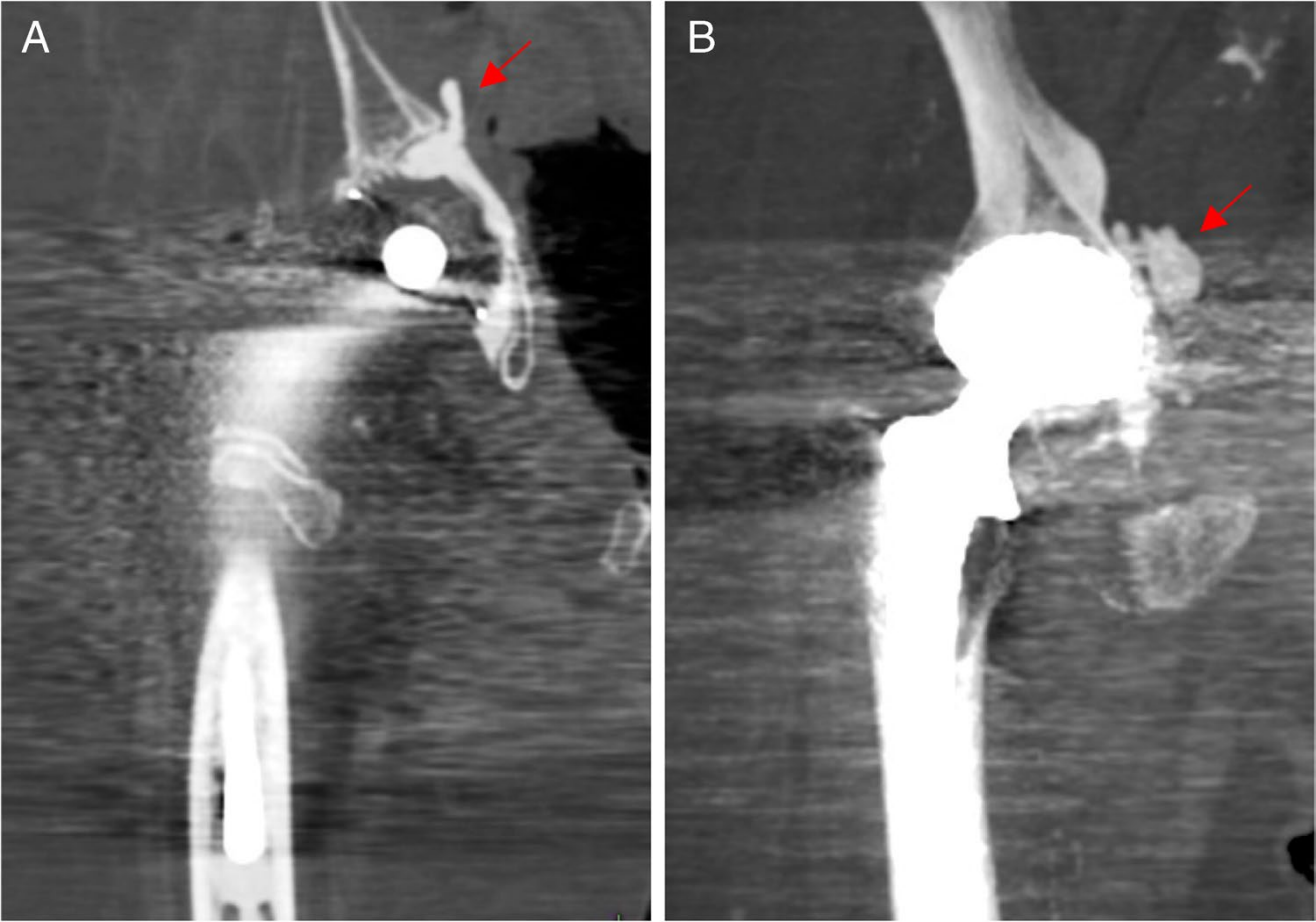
Examples of pelvic CT images with local periprosthetic cement leakage. A Needle-shaped cement leakage into the small basin. B Oval cement leakage into the small basin. The red arrows point to the cement leakage
